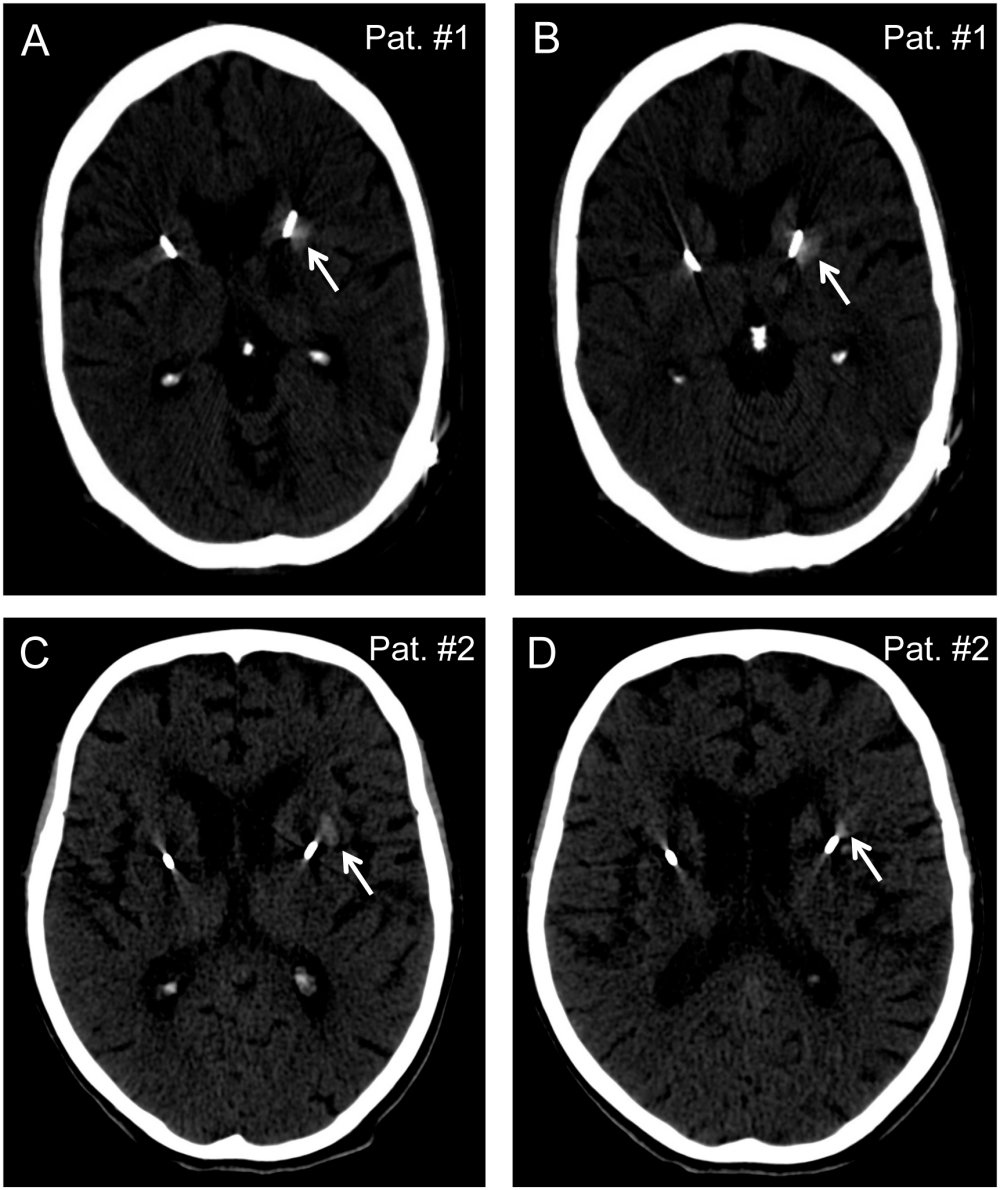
Intracerebral hemorrhages in the striatum along implanted electrodes (arrows) as detected in CT scans obtained on postoperative day three (A and B, patient#1; STN stimulation; diameter 1.2 cm) and day one (C and D, patient#2; GPI stimulation; diameter < 1 cm).
